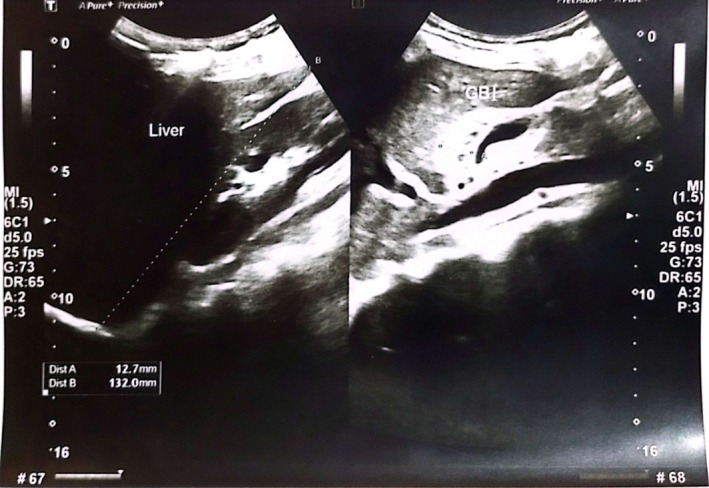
Abdominal ultrasound showing a normal‐sized liver with altered echogenicity and characteristic “starry sky” appearance. The hyperechoic portal triads with periportal edema and reduced background hepatic echogenicity are indicative of acute hepatitis changes.
